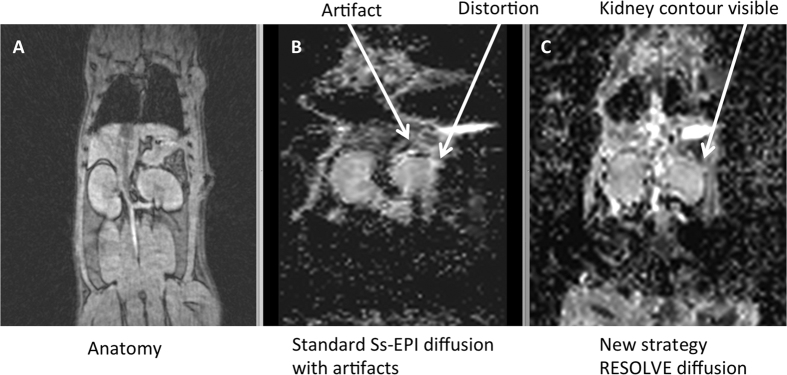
Comparison between single-shot (ss-EPI) and RESOLVE DWI MR sequences in a
